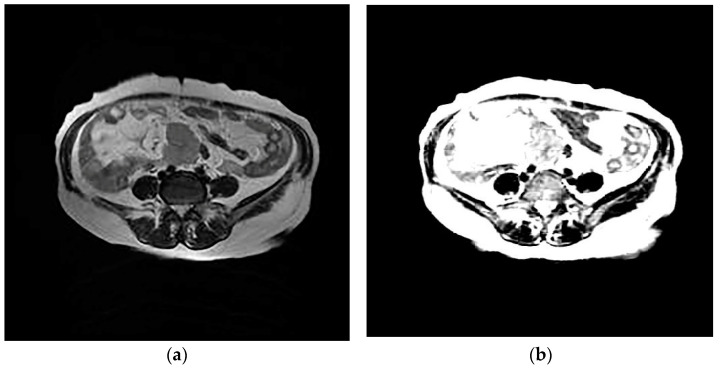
Axial T2-weighted image (a); generated image (b).
